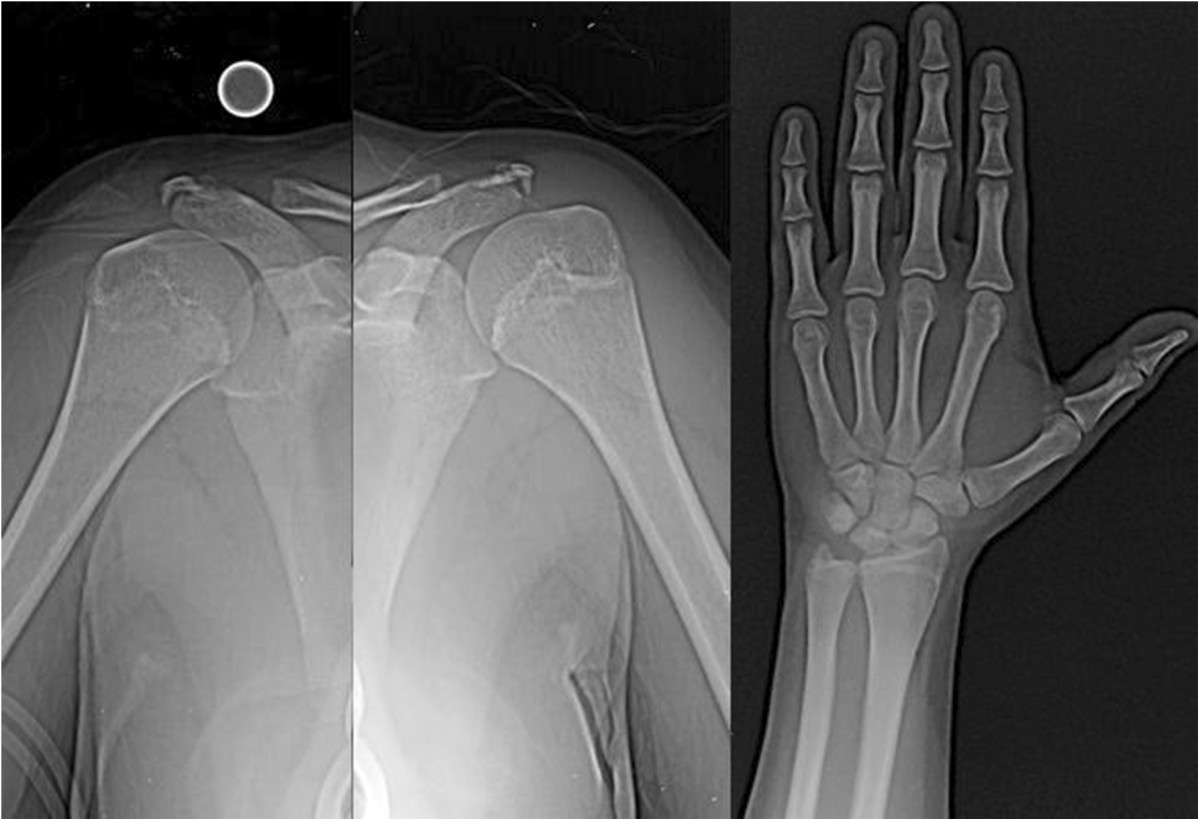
Anteroposterior radiographs of both shoulders and the right wrist, where the presence of open physis can be observed.
